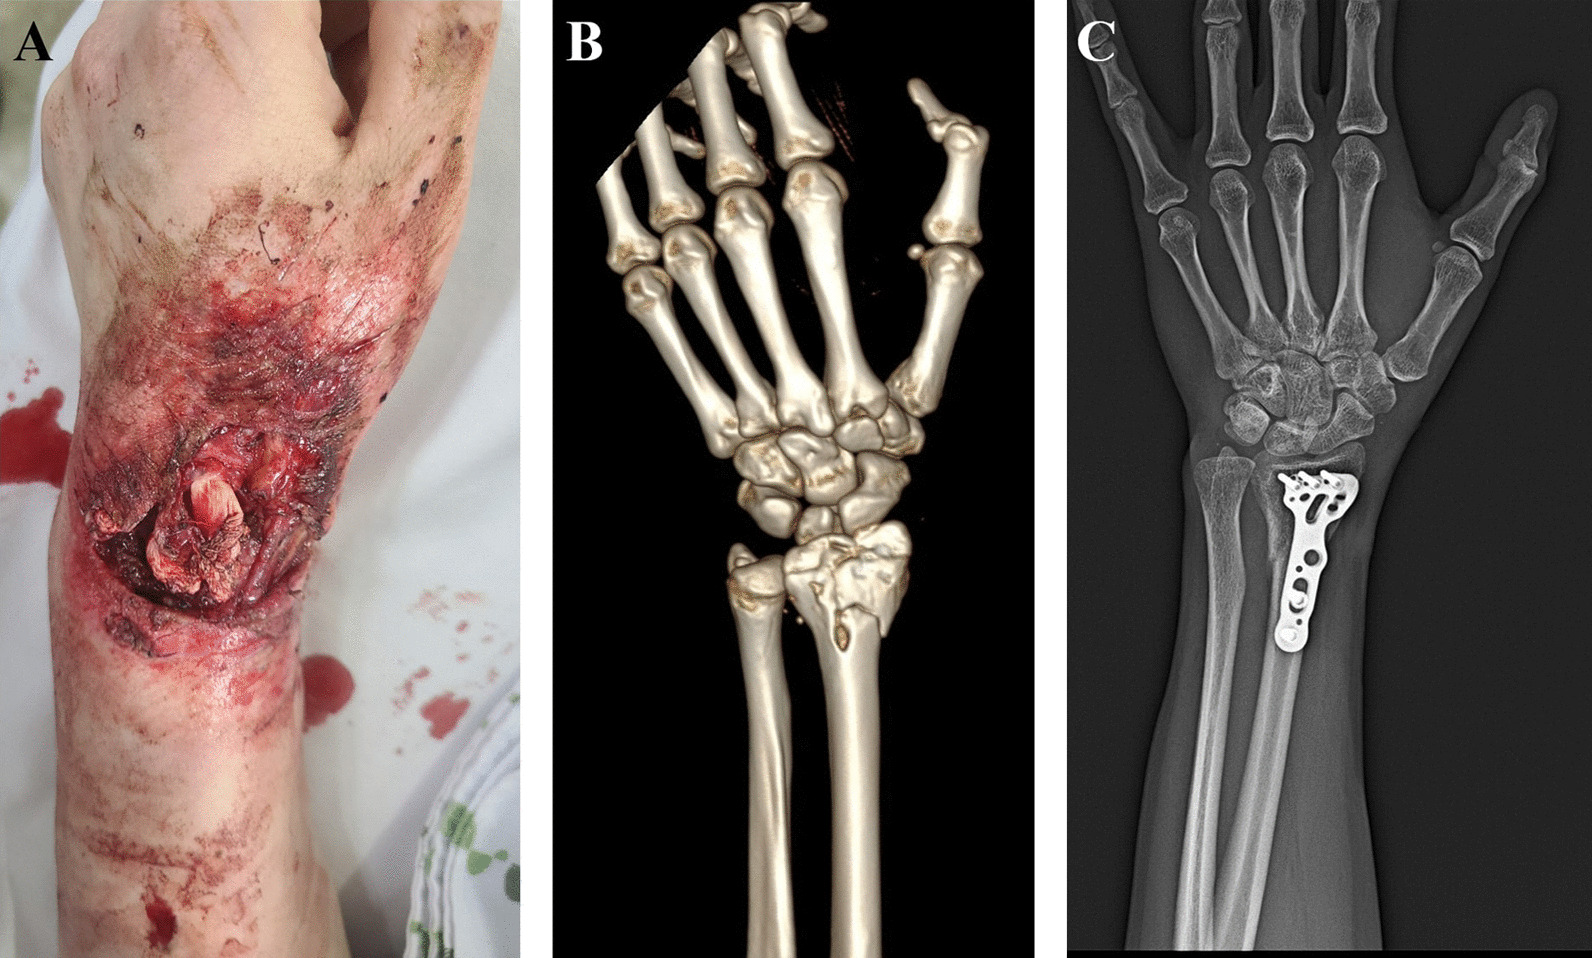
A 41-year-old woman with left wrist wound. A Initial wound. B CT scan of the fractured bone. C X-ray after internal fixation
